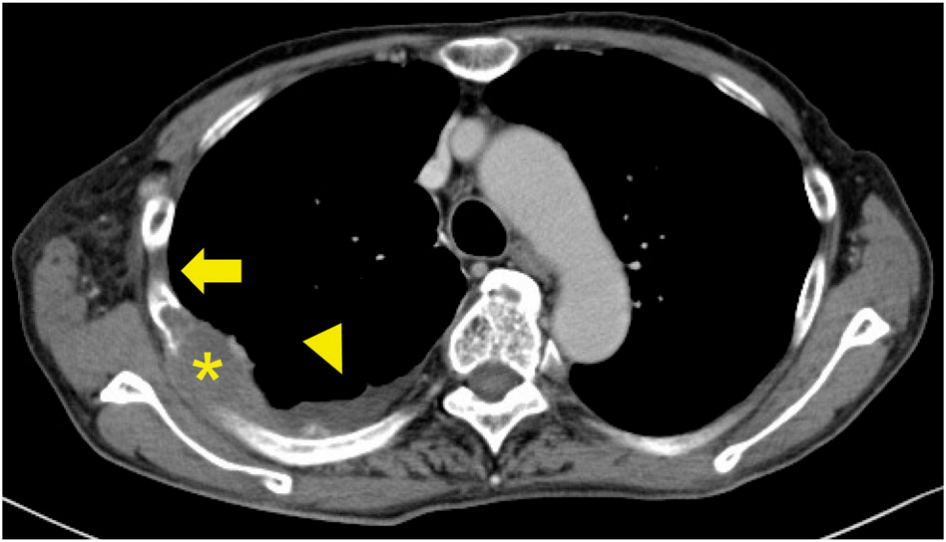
Computed tomography images after treatment. Thoracic SMARCA4‐deficient undifferentiated tumor showing osteolytic changes in the ribs (asterisk) is noted. However, pleural thickening (yellow arrow) disappears and pleural effusion (yellow arrowhead) decreases in the mediastinal window setting.
